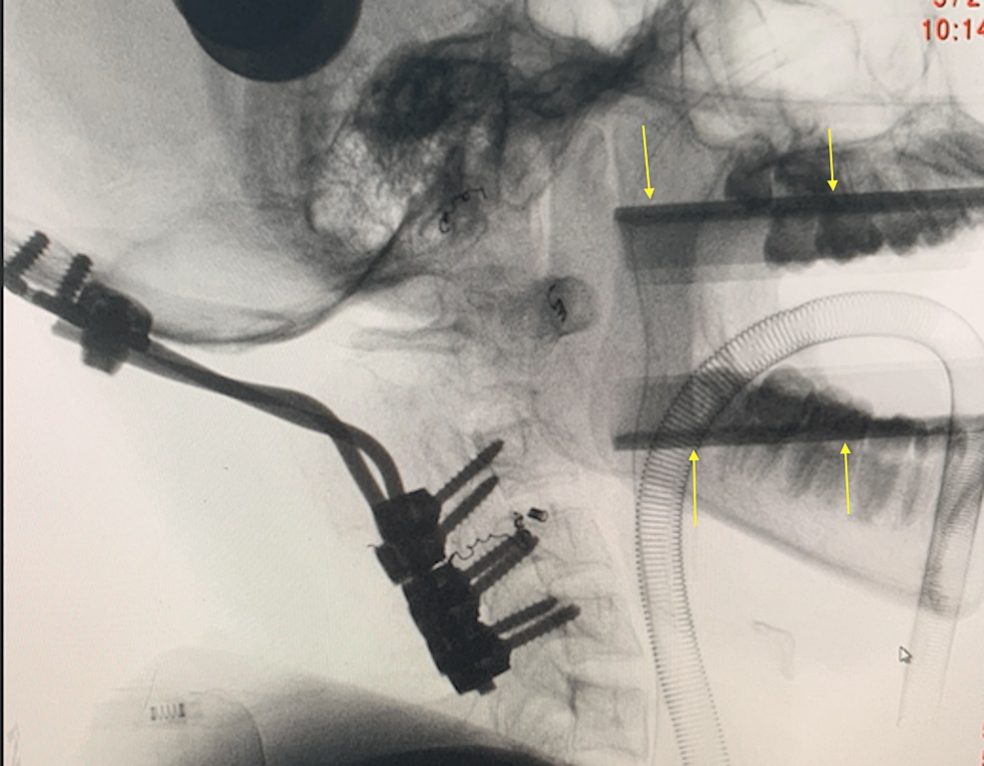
Intra-operative radiography showing access to C2 vertebral body Intra-oral retractors (yellow arrows) are used to visualize the retro-pharyngeal wall for access to the C2 vertebral body.
